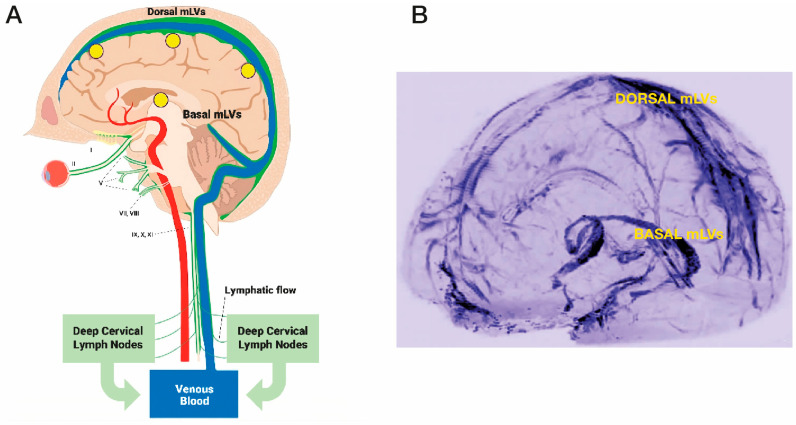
(A) Meningeal lymphatic vessels (mLVs), located within the brain’s meninges/dura, consist of both “Dorsal” and “Basal” lymphatic components (green vessels). Note the close parallel relationship of mLVs to venous sinuses (blue) within the brain. Selected cranial nerves are indicated with roman numerals. (B) Visualization of Dorsal and Basal meningeal lymphatic vessels in human brain using high-resolution Magnetic Resonance Imaging with Gadobutrol contrast. CSF within mLVs is first transported to cervical lymph nodes and then into the venous circulation. Yellow circles in (A) depict the approximate head surface locations on the left side of the head for the four radiofrequency emitters of a MemorEM device (see Figure 2B,C). Figure 2A reproduced from Arendash [106] and Figure 2B adapted from Absinta et al. [107].
